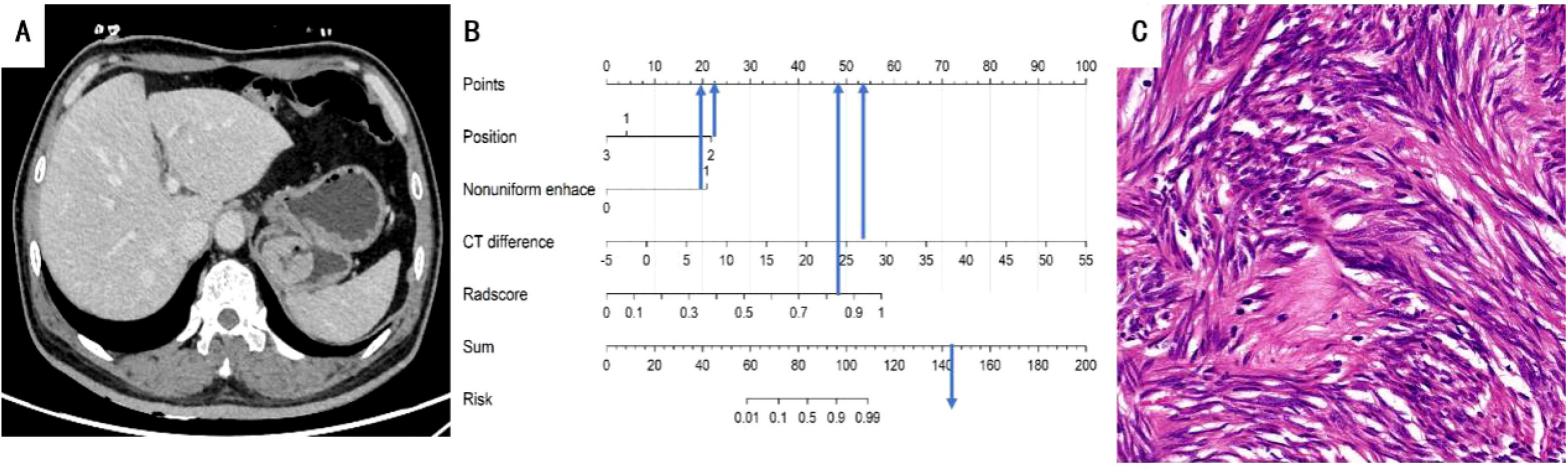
(A) A 63-year-old man with GIST. The axial CT image demonstrated a 3.8-cm-sized tumor in the fundus of the stomach. Axial CT image showed mucosal ulceration at the margin of the mass. (B) Total points of the tumor were 144, risk value was 1.6 and it was classified as GIST by the nomogram. (C) Pathological HE (×200) of the tumor.
